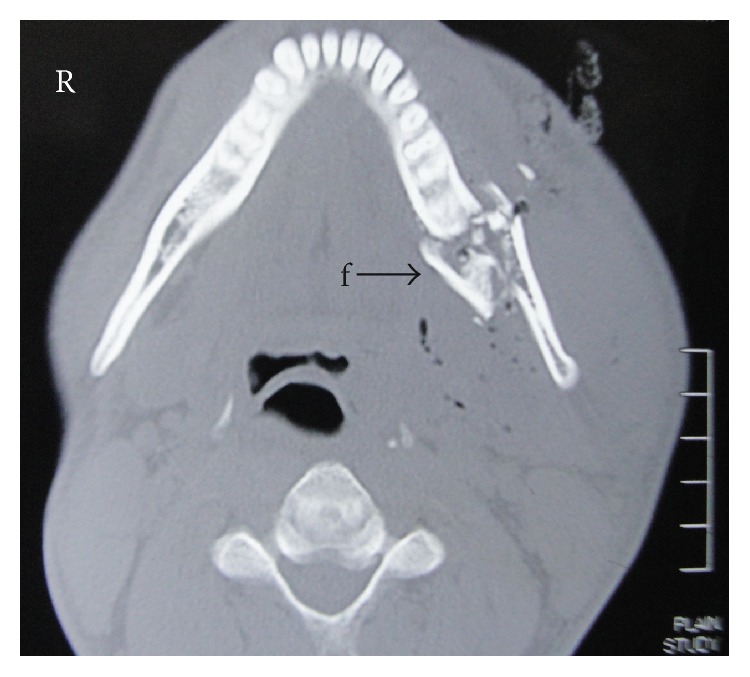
Axial CT scan showing multiple fractures of the mandible with inward displacement.
